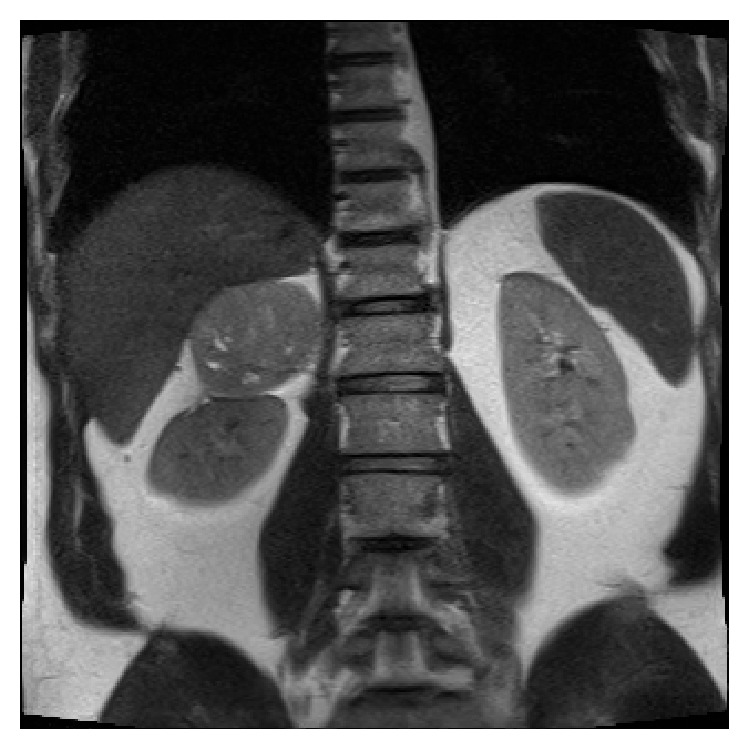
Coronal section of magnetic resonance imaging (MRI) showing 7.3 cm right adrenal mass.
